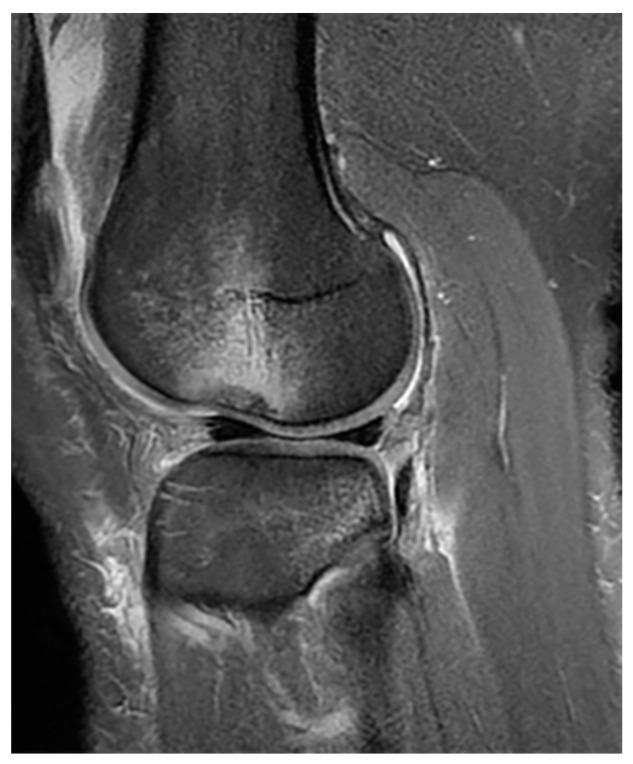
Lateral femoral notch sign on sagittal T2-weighted magnetic resonance imaging in an anterior cruciate ligament injury.
